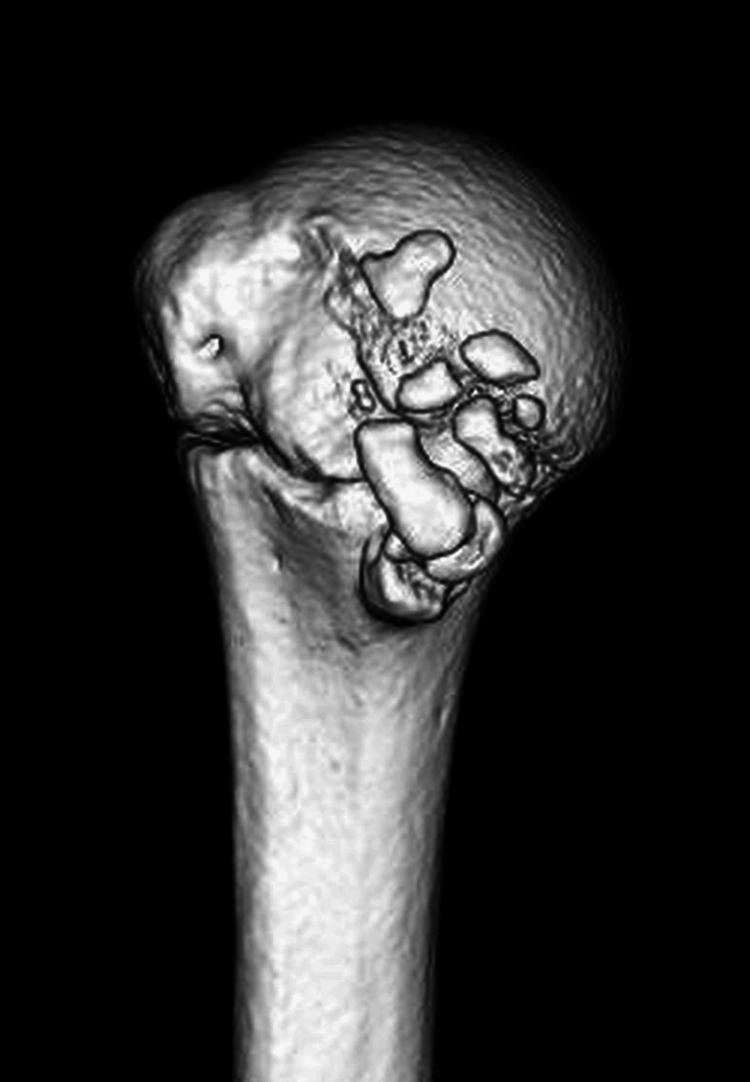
CT revealing multiple fragments at the lesser tuberosity CT: computed tomography
